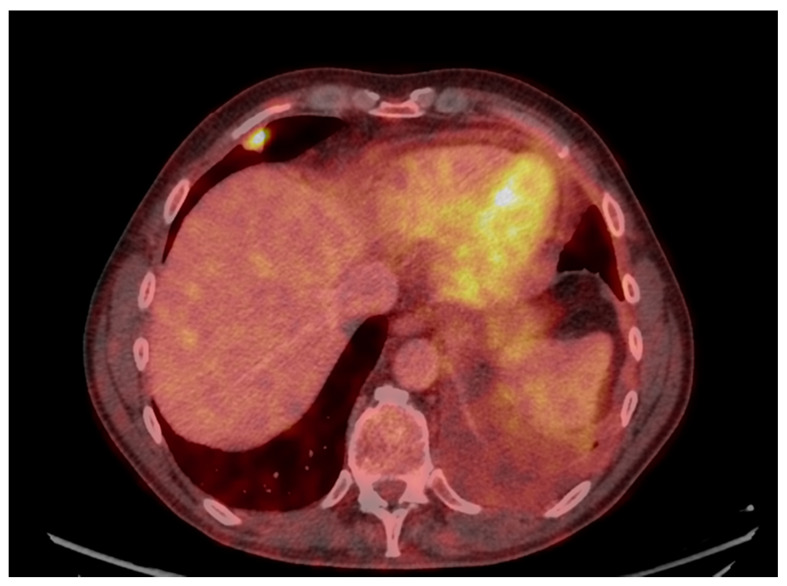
FDG-PET/CT showing pleural metastasis in the right lung’s middle lobe.
